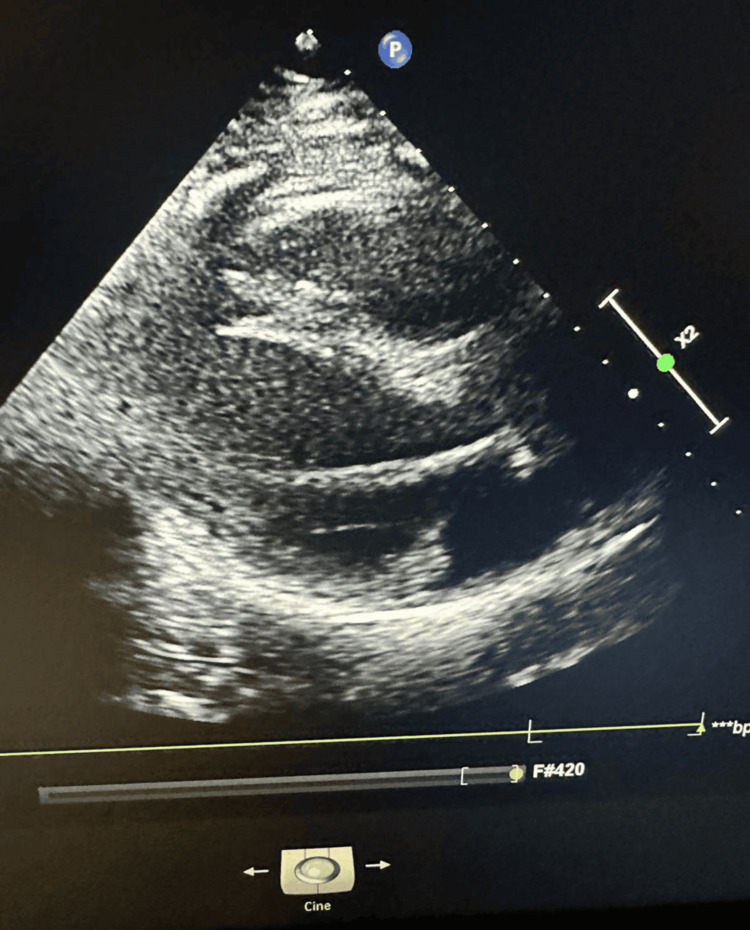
Follow-up transthoracic echocardiography showing normalized left ventricular systolic function (55%) with complete resolution of wall motion abnormalities
